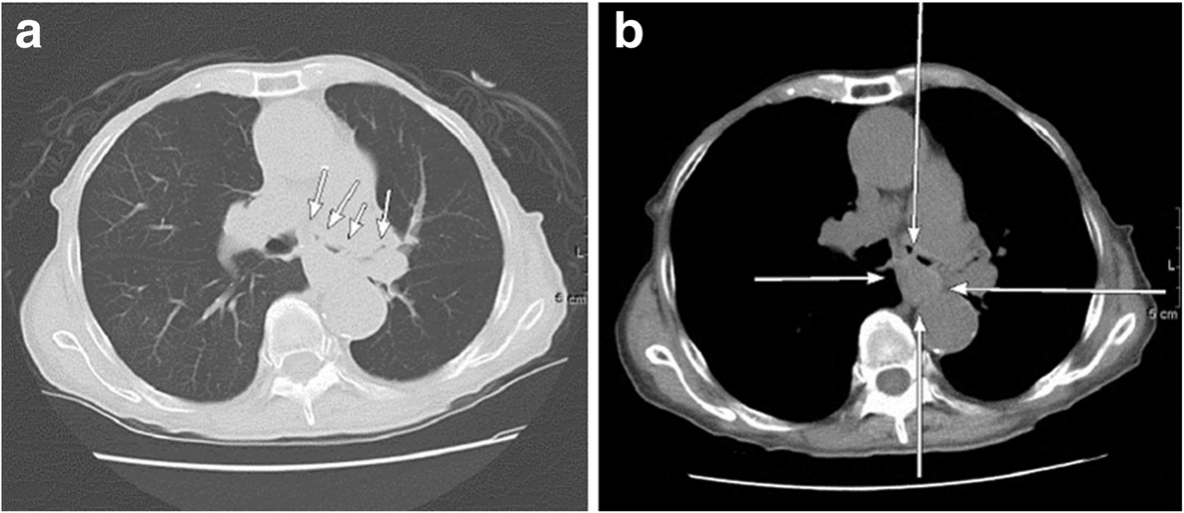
Mediastinal computed tomography showed a mass lesion on the middle esophagus that had grown extraluminally and had infiltrated the tracheal bifurcation and the left primary bronchus (a, b).
